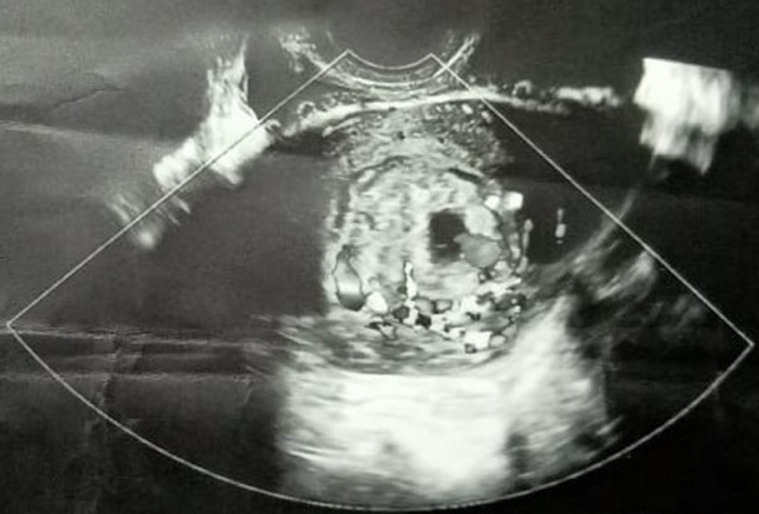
Doppler study showing vascular changes
